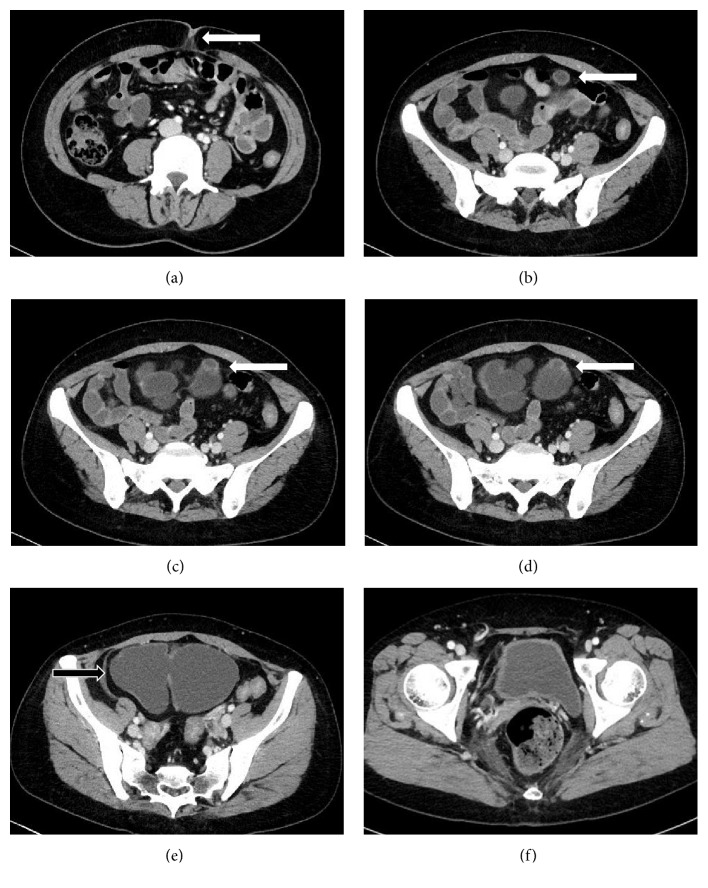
Abdominal CT shows urachal remnant and bladder diverticulum. (a–d) The fibrous cord runs from the umbilicus to the dome of the bladder (arrow). (e) A large bladder diverticulum locates in the right lateral wall (arrow). (f) CT findings nine months after the operation revealing no recurrence of the diverticula.
